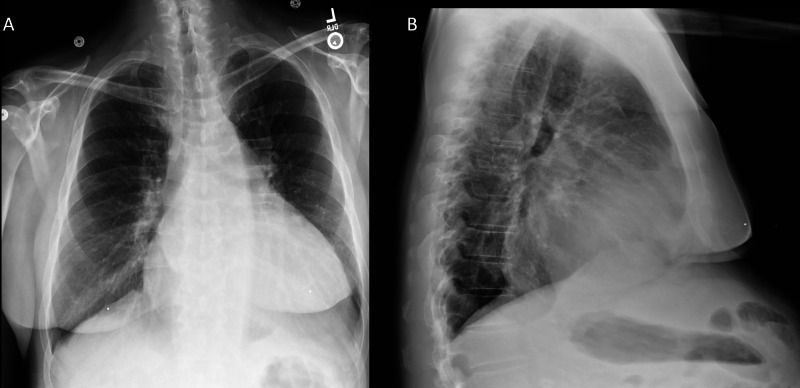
Chest X-Ray (A) Posterior-anterior chest X-ray with clear lung fields. (B) Lateral chest X-ray with clear lung fields.
